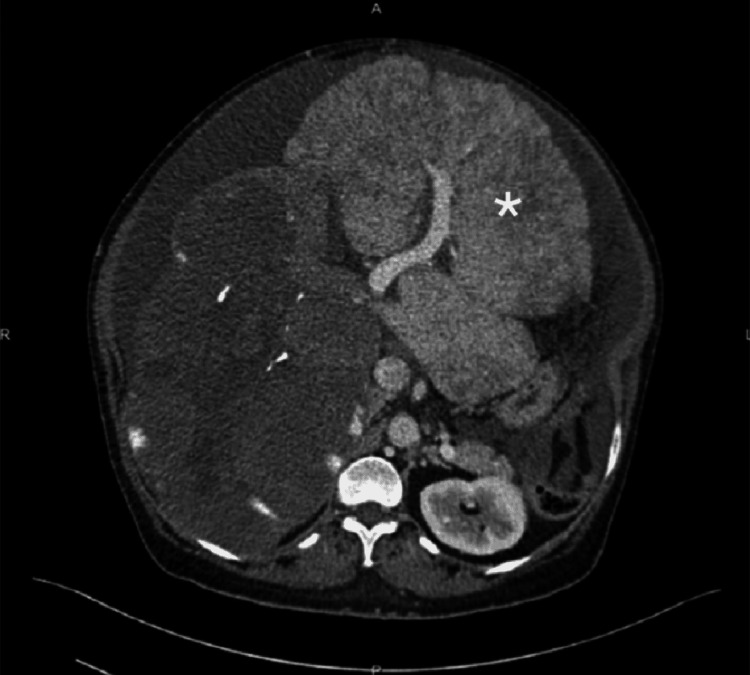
Axial non-contrasted CT image of the liver showing a giant fungating hemangioma (star).
